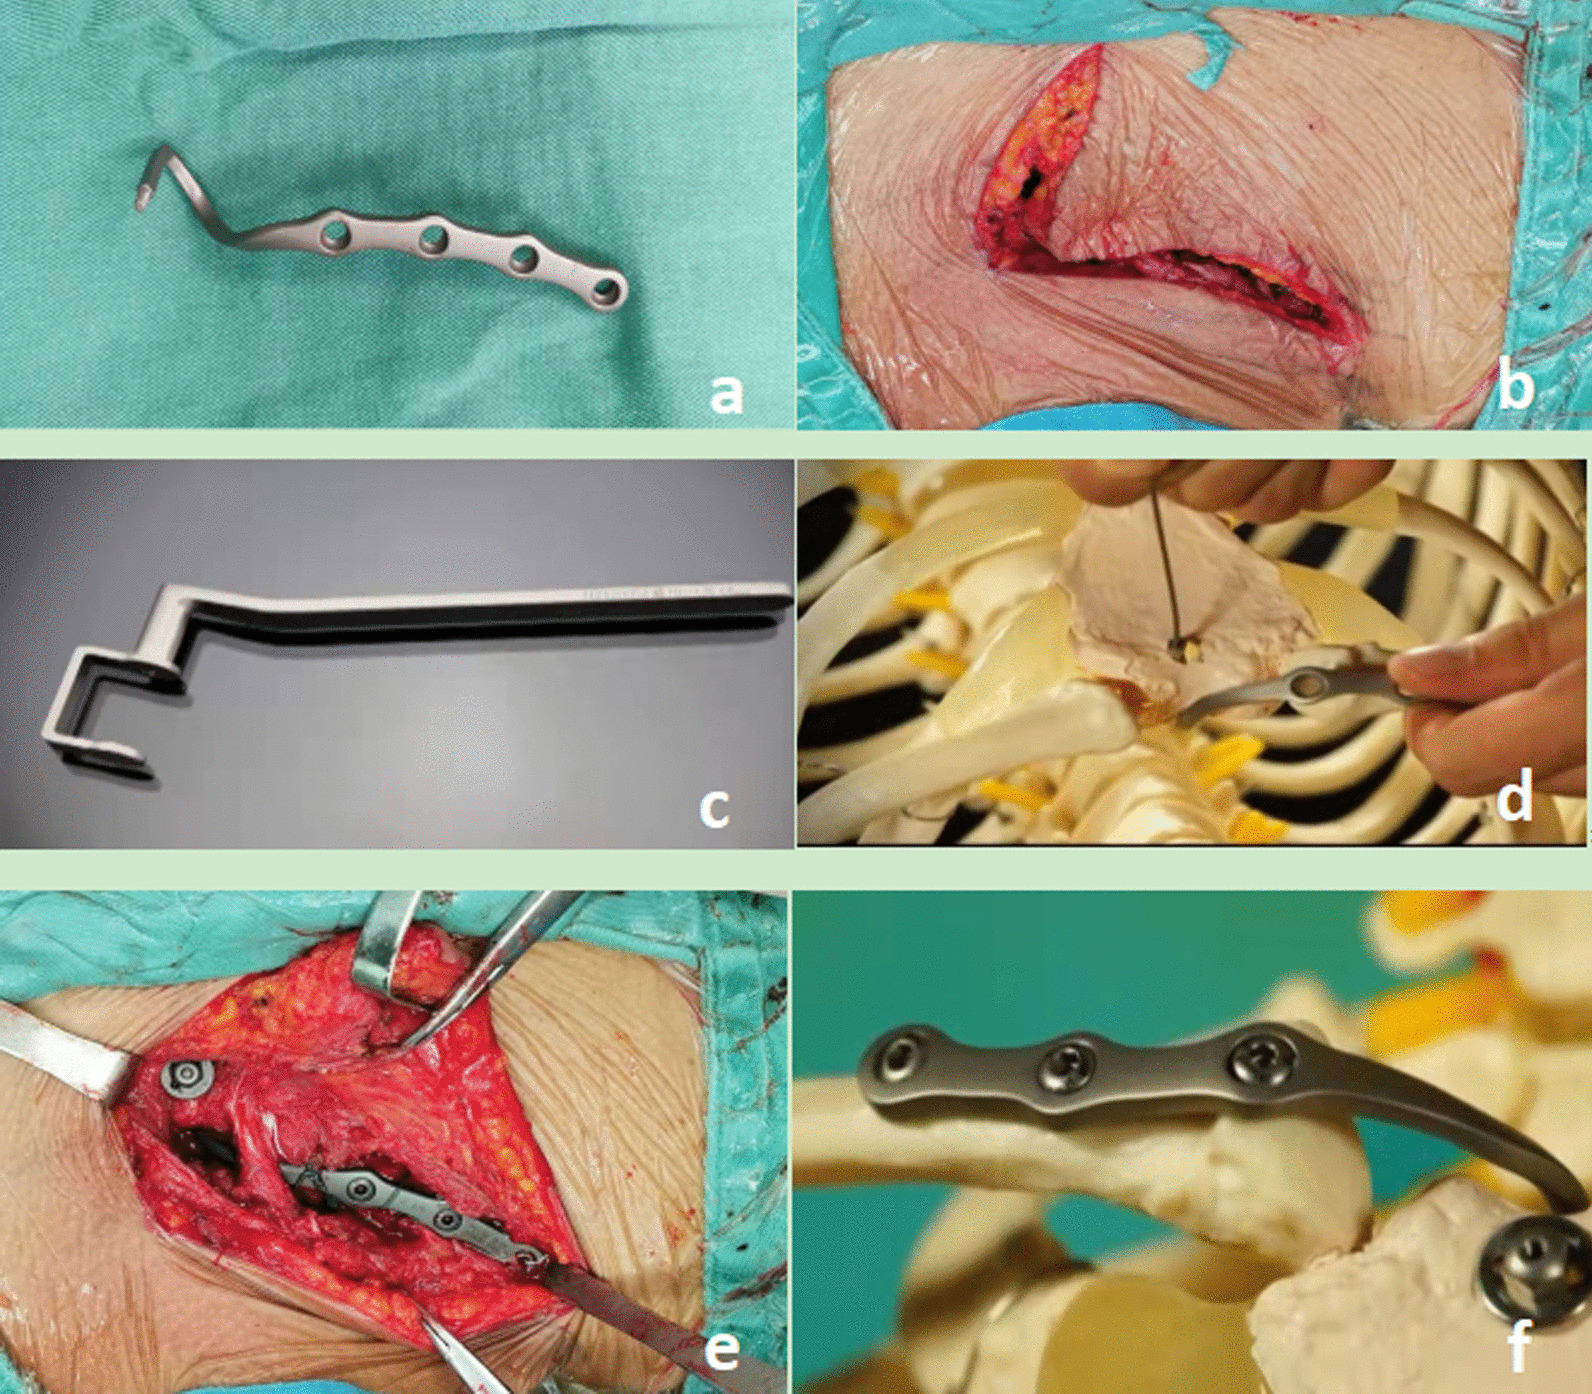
a, b Preoperative CT scan image show a right anterior dislocation of the sternoclavicular joint and proximal clavicle fracture, c Preoperative three-dimensional CT scan image, d Proximal end of the clavicle was displaced anteriorly and proximal clavicle fracture
